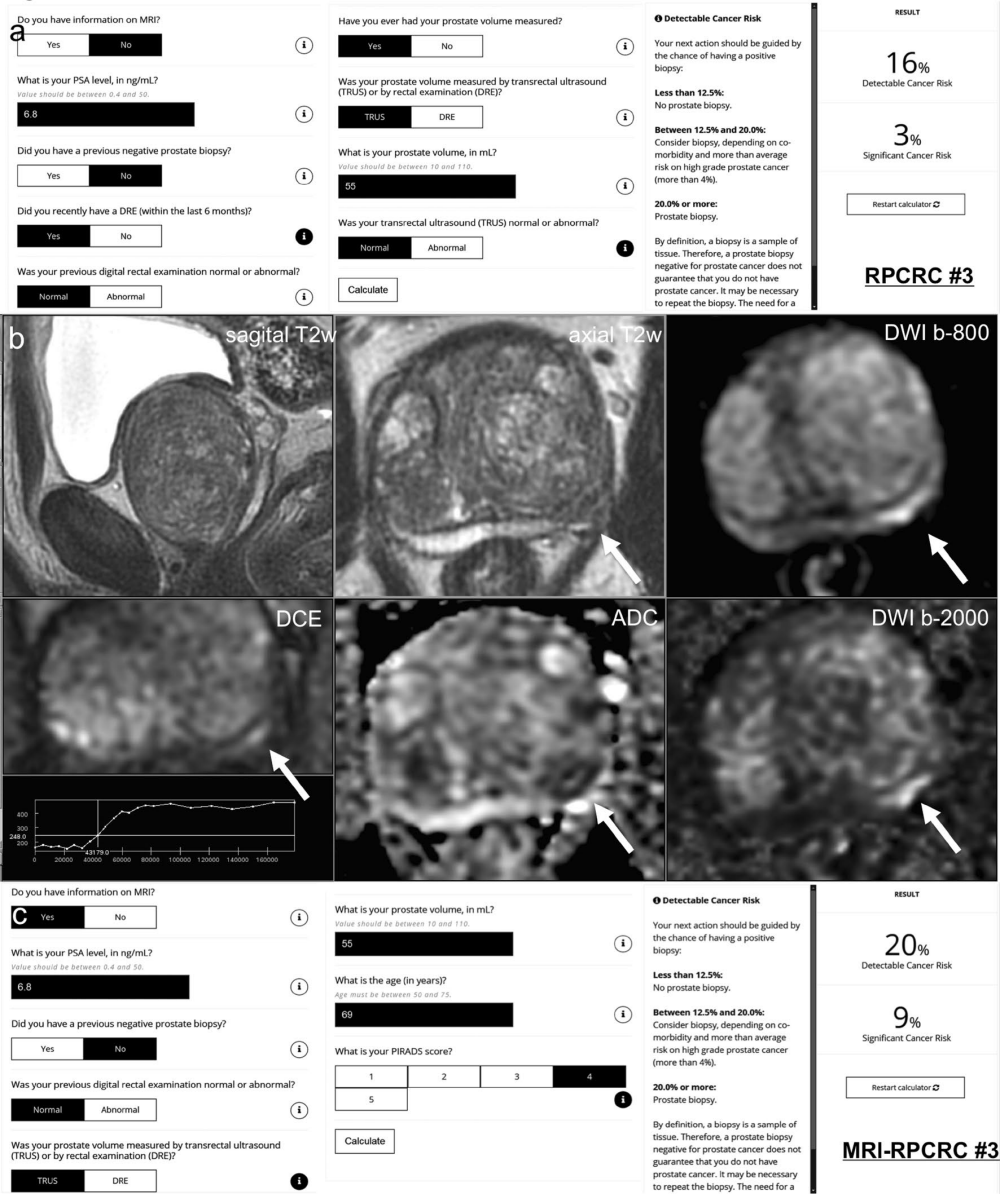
Case example: a 69-year-old man with a PSA of 6.8 ng/ml. Digital rectal examination (DRE) was normal, and transrectal ultrasound (TRUS) showed a prostate volume of 55 ml, without any hypo-echoic lesions found on ultrasound. The PSA density was 0.12 ng/ml^2^ (6.8/55). *Does this biopsy-naive man deserve an MRI and/or biopsy?* (1) In this biopsy-naïve man, the chance of finding any prostate cancer with further testing is 32%, based on the *ERSPC-RC#2* (including PSA only in biopsy-naïve men) [49], suggesting a systematic biopsy is justified. (2) The chance of finding any prostate cancer and clinically significant prostate cancer with further testing is 16% and 3%, respectively, based on the *ERSPC-RC#3* (including PSA, DRE, TRUS, and TRUS volume) [49] (a). Including DRE, TRUS, and TRUS volume in the risk calculation gives a lower risk estimation of having a biopsy-detectable clinically significant prostate cancer. The next action should be guided by the chance of having a positive biopsy: (1) less than 12.5% → no prostate biopsy (and no MRI); (2) between 12.5 and 20.0% → consider biopsy (and MRI), depending on co-morbidity and more than average risk on high grade prostate cancer (more than 4%); (3) 20.0% or more → prostate biopsy (and MRI). In this man, a systematic biopsy is justified, based on the ERSPC-RC#3. (3) A 3T multi-parametric MRI was performed (b), showing a large prostate gland with compression of the peripheral zone, and a suspected lesion for clinically significant prostate cancer at the left peripheral zone dorsolateral, at the mid-prostate, 12 × 4 mm, without extraprostatic extension or seminal vesicle invasion; PI-RADS assessment score 4 (4/4/+), based on low signal intensity on T2w-imaging (4), high signal intensity on DWI (b-800 and b-2000 (calculated) combined with low signal intensity on ADC (4), and focal enhancement (+). Based on high yields of clinically significant prostate cancer (> 30–40%) in an MRI lesion with a PI-RADS assessment score 4 (Sects. 3.2 and 4.2) [37], a targeted biopsy is indicated. (4) The chance of finding any prostate cancer and clinically significant prostate cancer with further testing is 20% and 9%, respectively, based on the *MRI-ERSPC-RC#3* (including PSA, DRE, TRUS, TRUS volume, and MRI PI-RADS score) [49] (c). In this man, a systematic and targeted biopsy is justified. *Biopsy considerations* the morphology of the suspected peripheral zone lesion is long and small; targeted cores of the lesions combined with targeted cores from the penumbra (focal saturation) could be sufficient. *Biopsy protocol and pathology findings* Protocolled 12 systematic biopsies and 2 targeted biopsies were performed under MRI/US fusion guidance. 12 systematic biopsies showed benign prostatic tissue only. 2 targeted biopsy cores showed Gleason score 3 + 4 (ISUP group 2) with 3 mm and 4 mm maximum cancer core length, without cribriform or intraductal growth pattern
